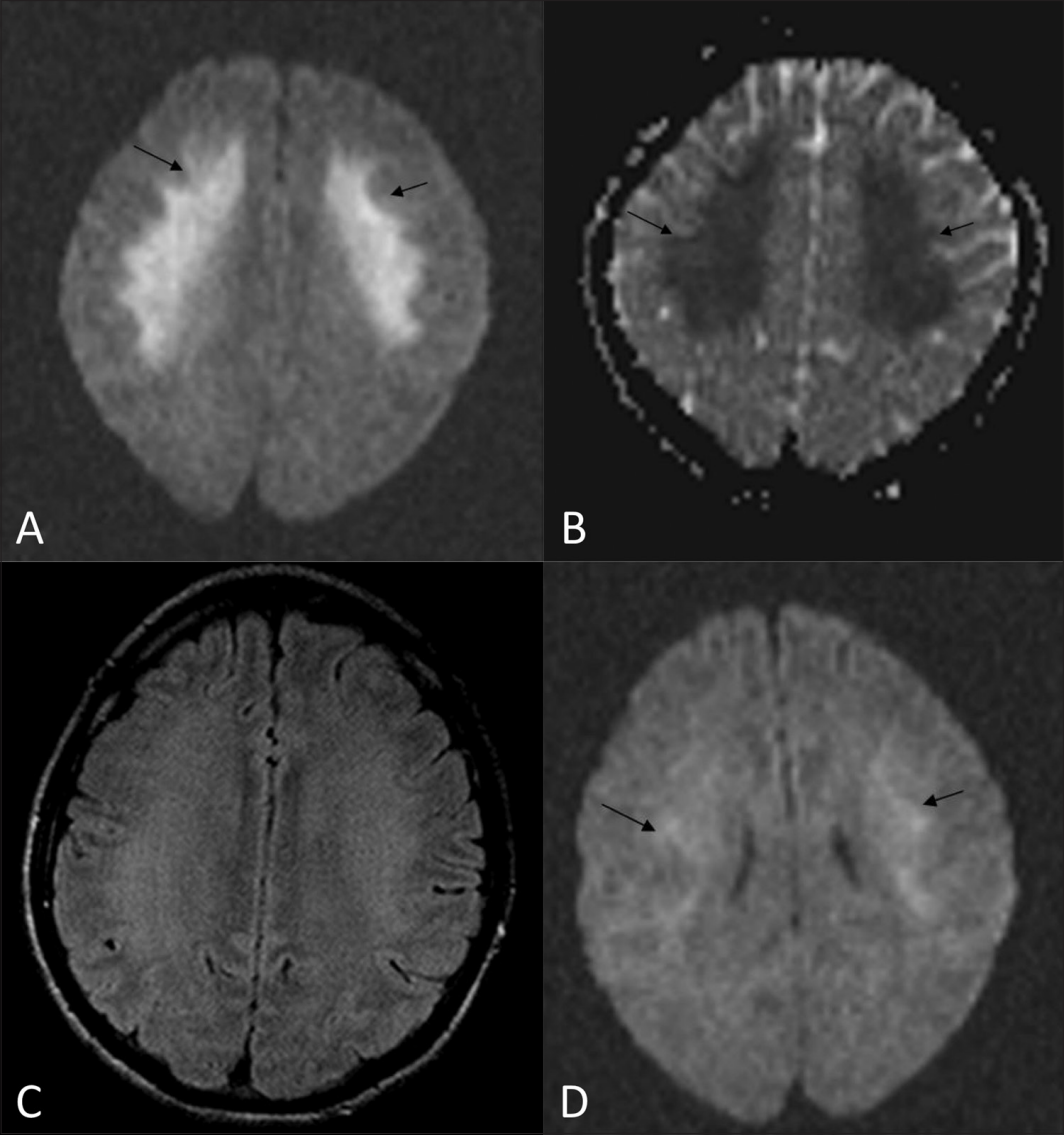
MRI. Axial diffusion-weighted (b1000) image (A) shows significant restriction of diffusion (arrows) in the frontal lobe white matter. The corresponding ADC image (B) shows decreased ADC, as evident by the dark signal (arrows) in the region of reduced diffusion (ADC: 350 – 380 10^-3^ mm^2^/s). Axial FLAIR image (C) shows no significant changes in the region of reduced diffusion. Follow-up MRI (diffusion-weighted imaging) after 3 days (D) shows resolution of the white matter changes
